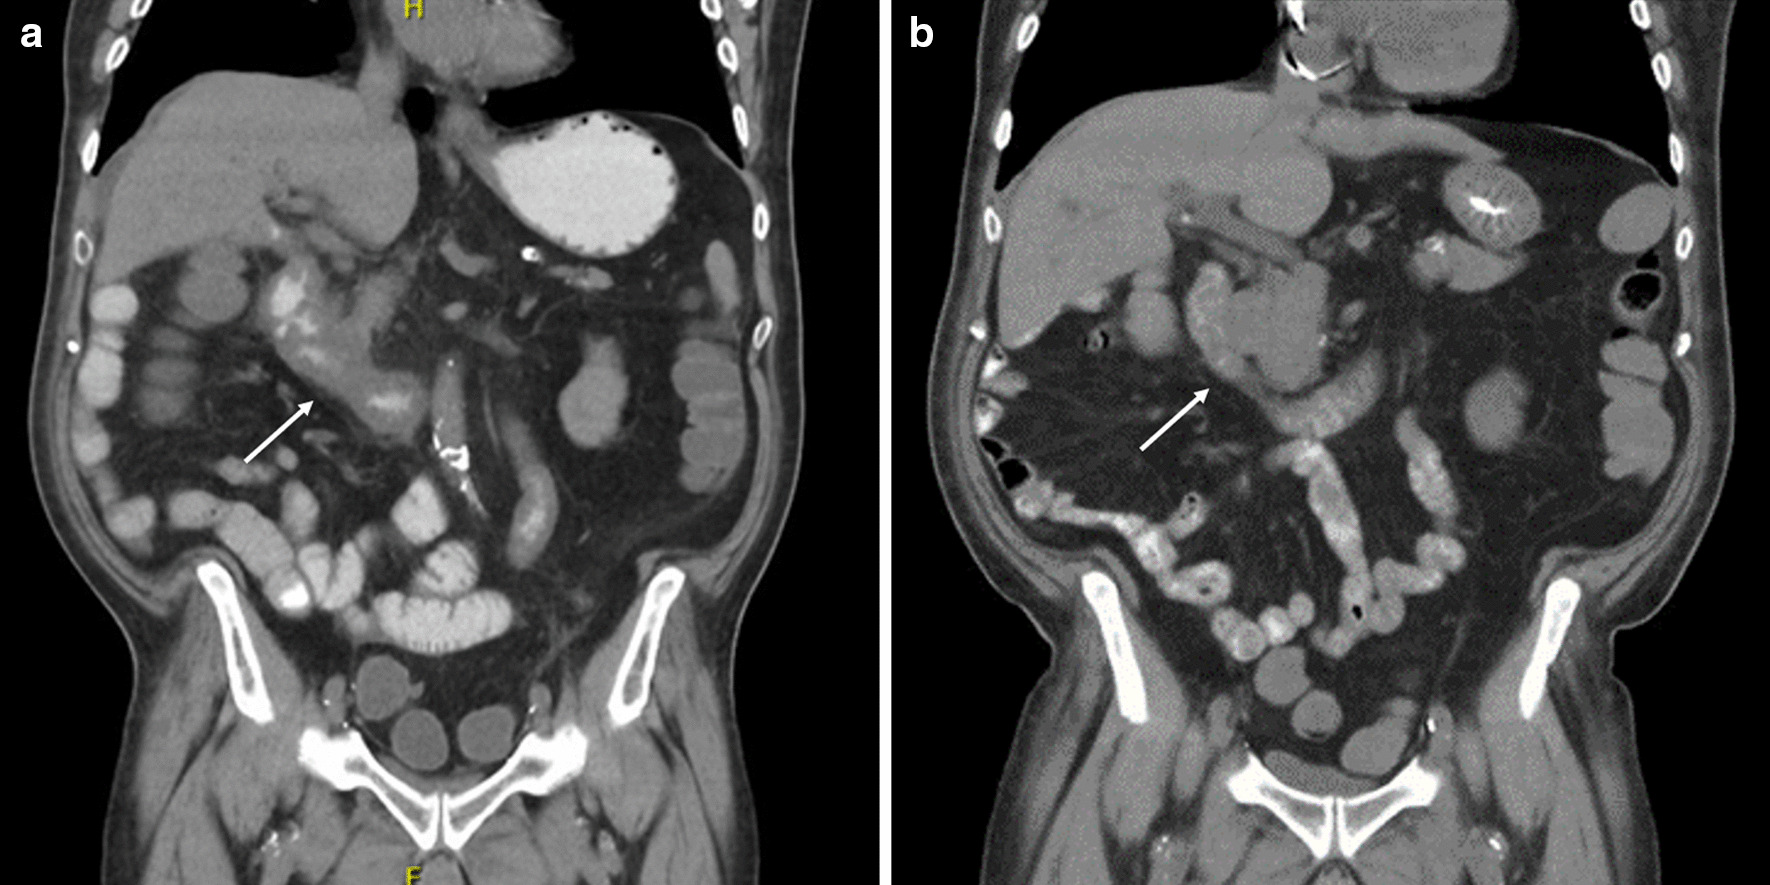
a CT abdomen/pelvis on initial presentation with enteritis and lack of colitis. b CT abdomen/pelvis on second presentation with hemorrhagic shock, showing improved enteritis
